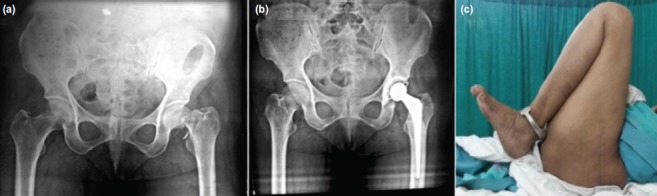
(a) Pre and (b) Post op radiographic images of patient treated with bipolar prosthesis for femur neck fracture. (c) Clinical picture of patient after one year of treatment.
